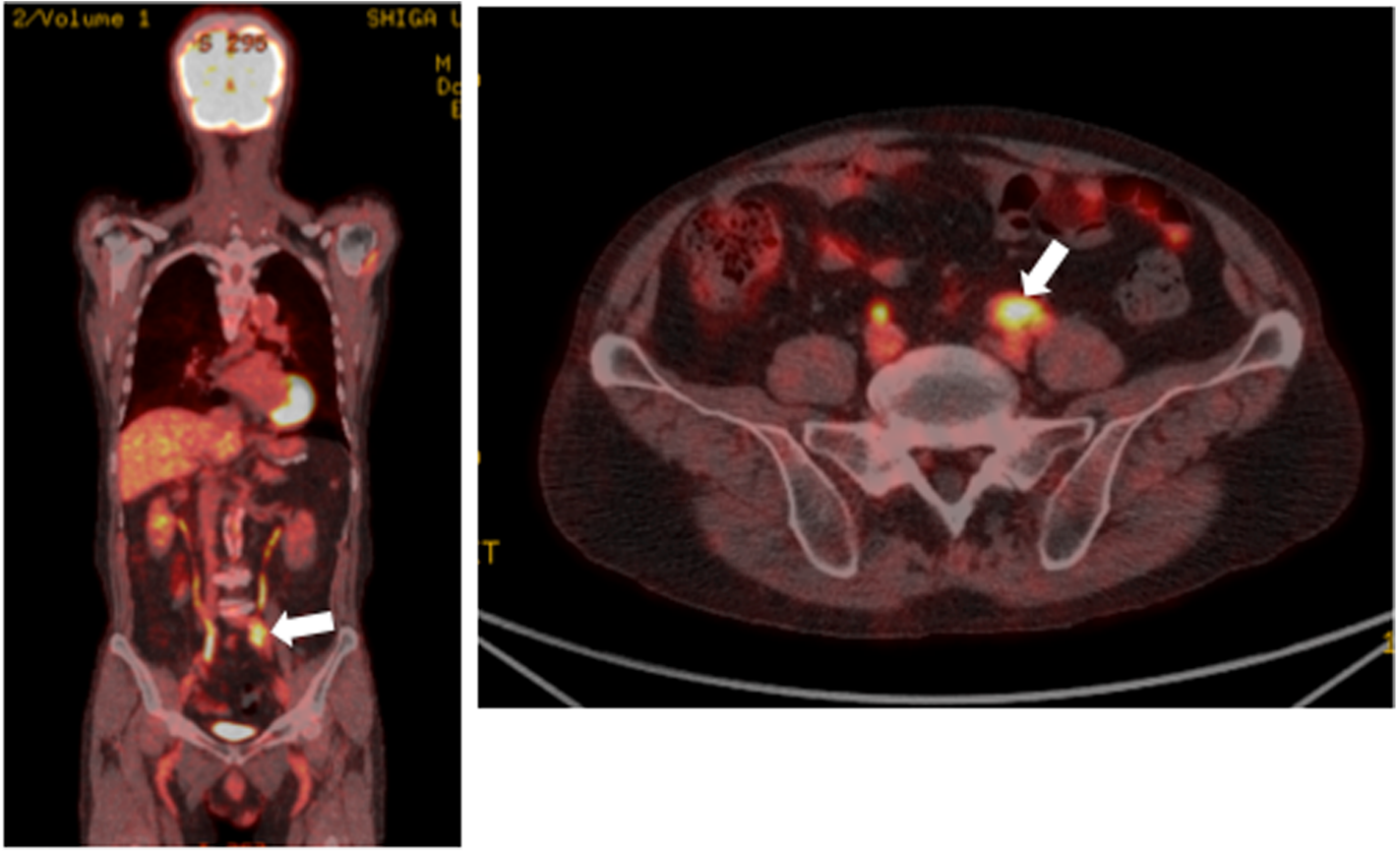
Fluorine-18 fluorodeoxyglucose (FDG) positron emission tomography CT (PET-CT) findings. FDG-PET-CT showed significant accumulation of FDG uptake in the same region (maximum standardized uptake value, 8.93; arrow)
